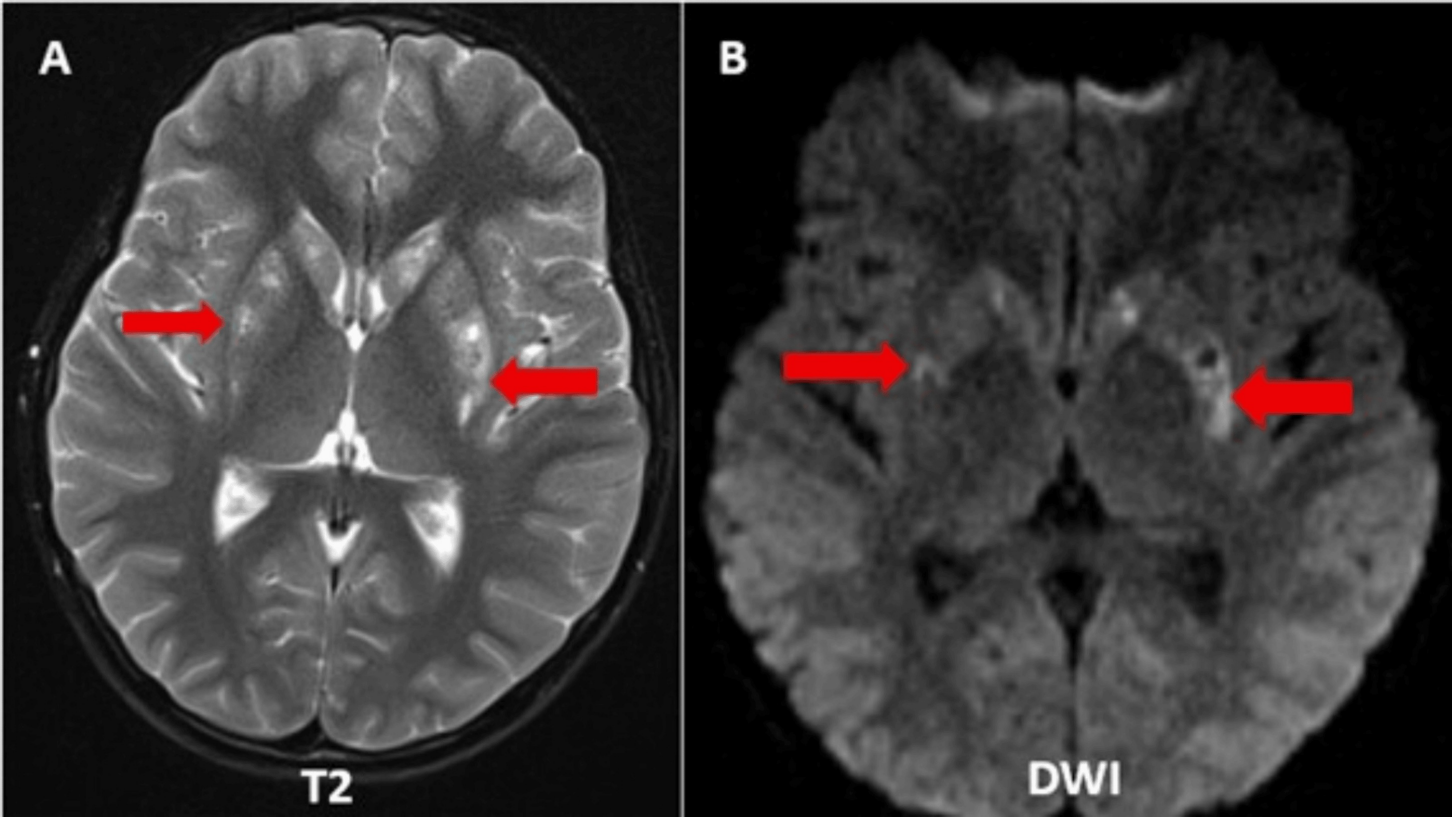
Axial sections of MRI brain (bilateral corpus striatum). (A) Multiple T2 hyperintense foci in bilateral corpus striatum (red arrows). (B) Restricted diffusion in the same areas (red arrows). MRI: magnetic resonance imaging; DWI: diffusion-weighted imaging
